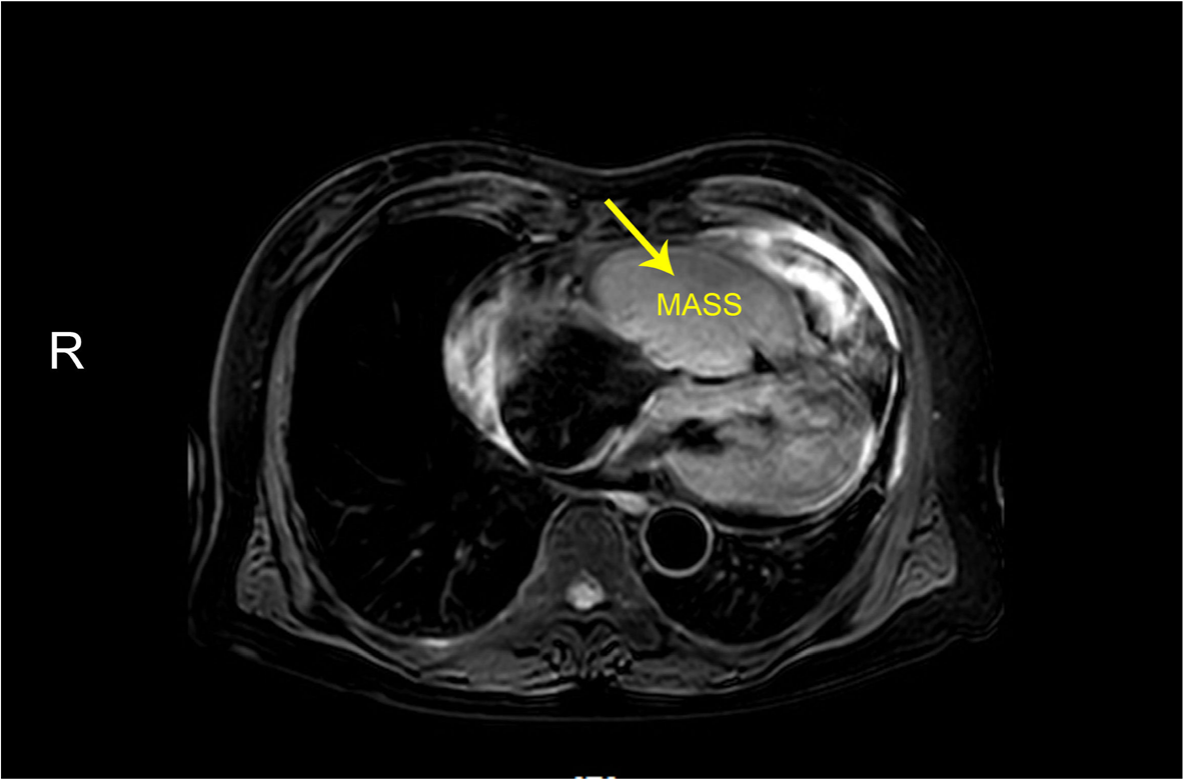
Cardiac MRI, T2 weighted image showed intracavity RV mass in lobular appearance, with blurred outline with myocardium
